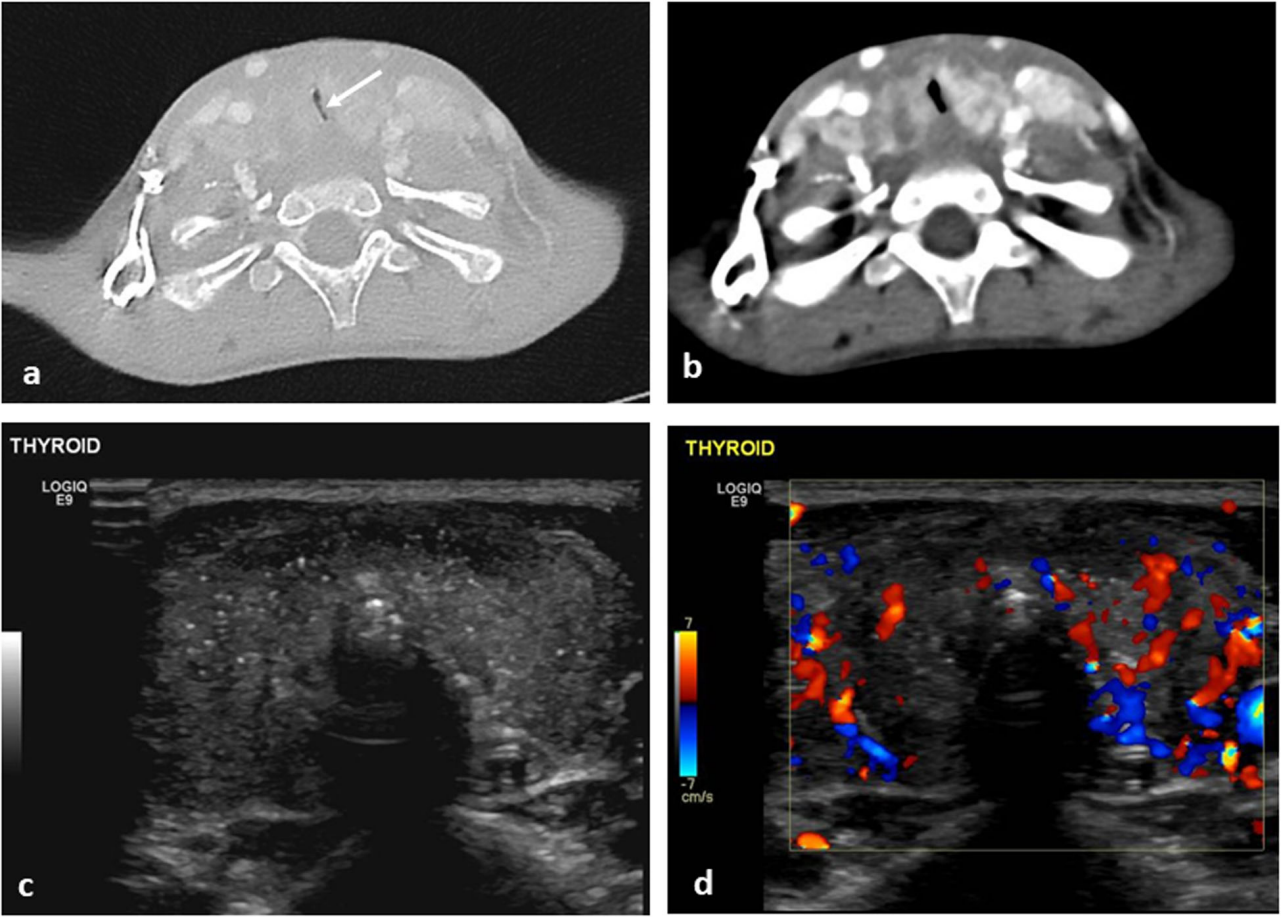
A 5-year-old boy with worsening stridor, dry cough and cervical lymphadenopathy with papillary thyroid carcinoma. a Axial CT of the neck (lung windows) demonstrates marked narrowing of the trachea, with slit-like appearance (arrow). b On the mediastinal windows, the thyroid gland is bulky and demonstrates patchy heterogenous enhancement. c Transverse ultrasound image of the thyroid gland prior to biopsy reveals multiple internal foci of calcification and (d) colour Doppler imaging reveals a hyper vascular thyroid gland
